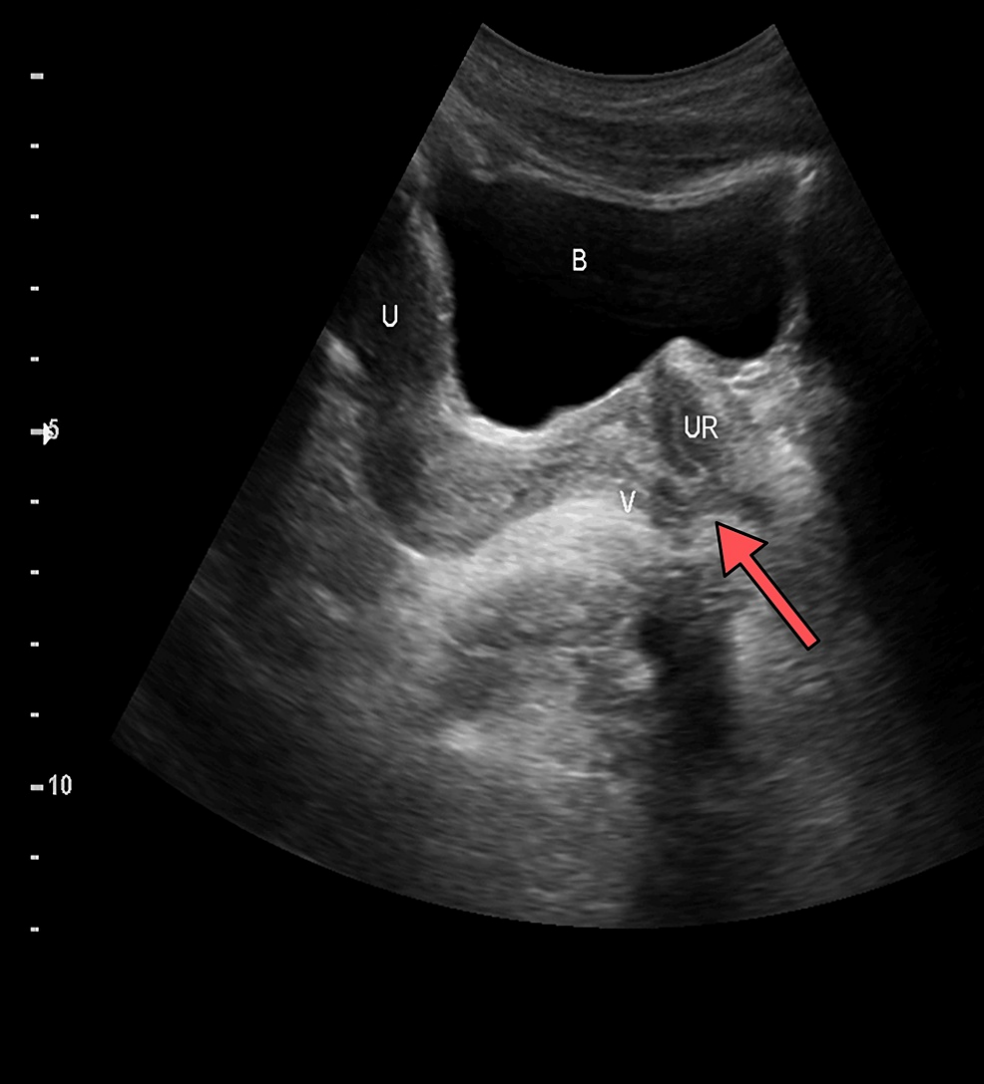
Ultrasound imaging of the pelvis revealing various structures. The abnormal connection between the lower part of the vagina and the posterior part of the urethra can be seen (red arrow). B: urinary bladder; U: uterus; V: vagina; UR: ureter
